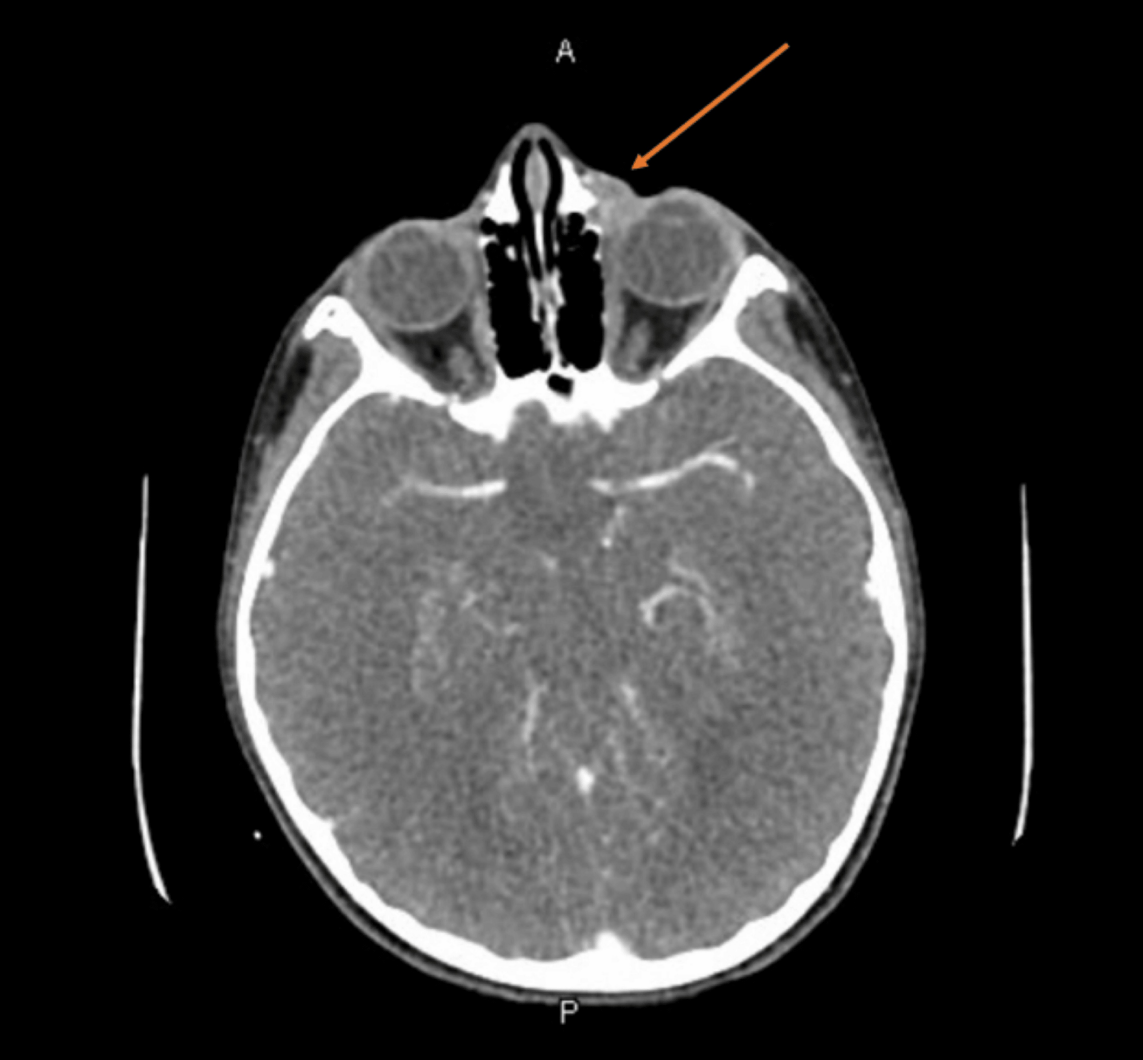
CT of the orbits with contrast The arrow points to the area of opacification commented on by radiology as dacryocystitis.
